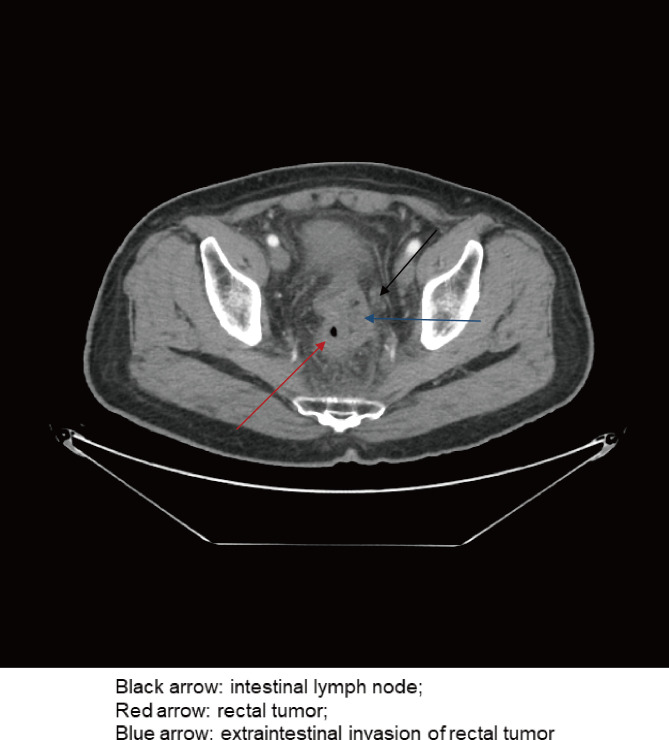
Post-neoadjuvant pelvic CT. The red arrow indicates the rectal tumor mass. The blue arrow points to suspected extramural invasion involving the perirectal fat. The black arrow shows an enlarged pararectal lymph node, suggesting persistent nodal disease (N2) despite neoadjuvant therapy.
